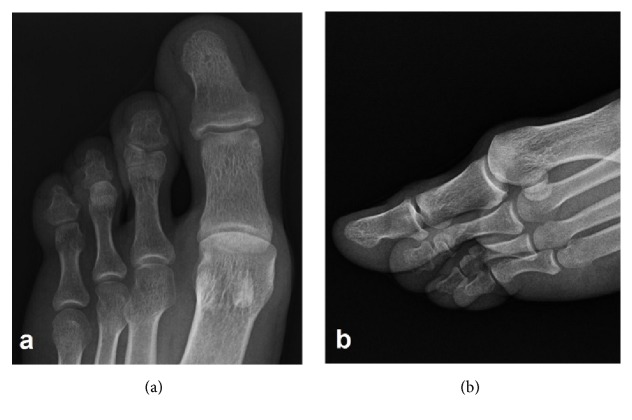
Magnified anteroposterior (a) and lateral oblique (b) radiographs of the left foot show that the middle phalanges of the second and third fingers are shorter than the distal phalanges, and the middle phalanx of the fourth finger is absent.
